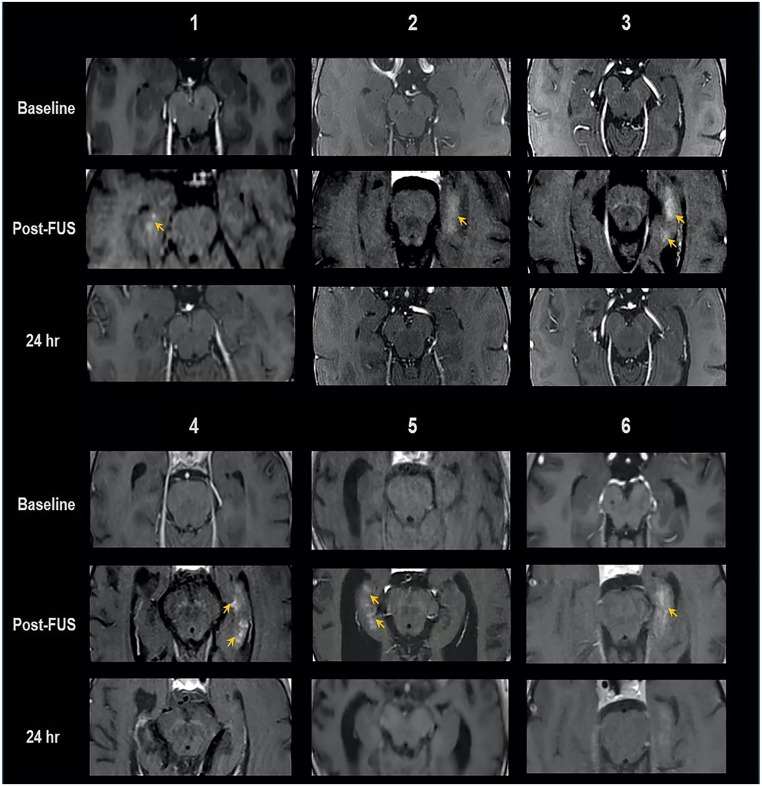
MRI evidence of BBB opening and closure in six subjects. Baseline, immediately post-FUS, and 24-h post-FUS contrast-enhanced T1 axial MRI shows parenchymal contrast enhancement at targeted sites (arrows), indicating BBB opening, and resolution at 24 h, following repeat contrast administration, indicative of BBB closure.
